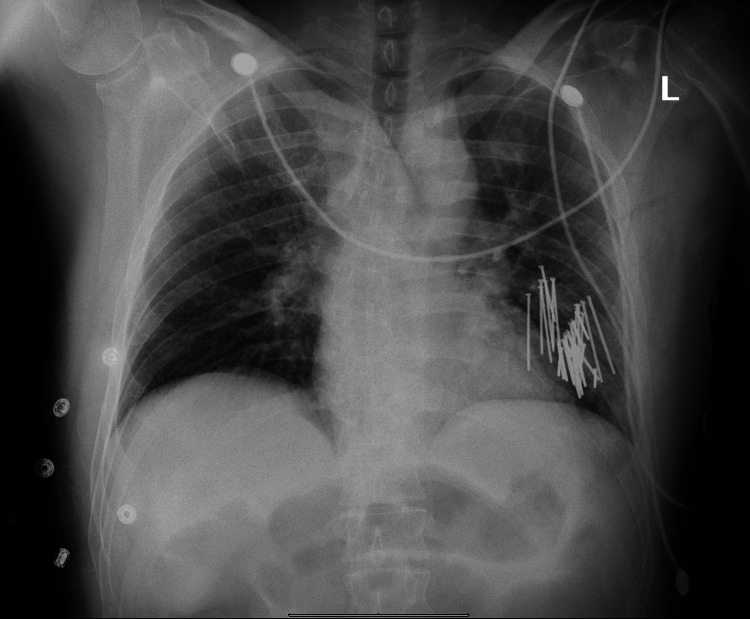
Chest x-ray upon initial presentation
